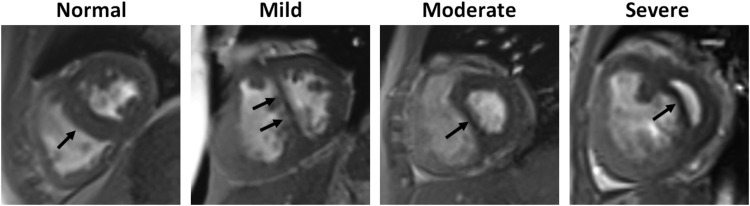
Septal wall bending in pulmonary arterial hypertension (PAH). Basal short-axis cine MR images at early diastole showing normal septal wall shape (left) as well as mild, moderate, and severe leftward septal wall bowing at different PAH stages.
